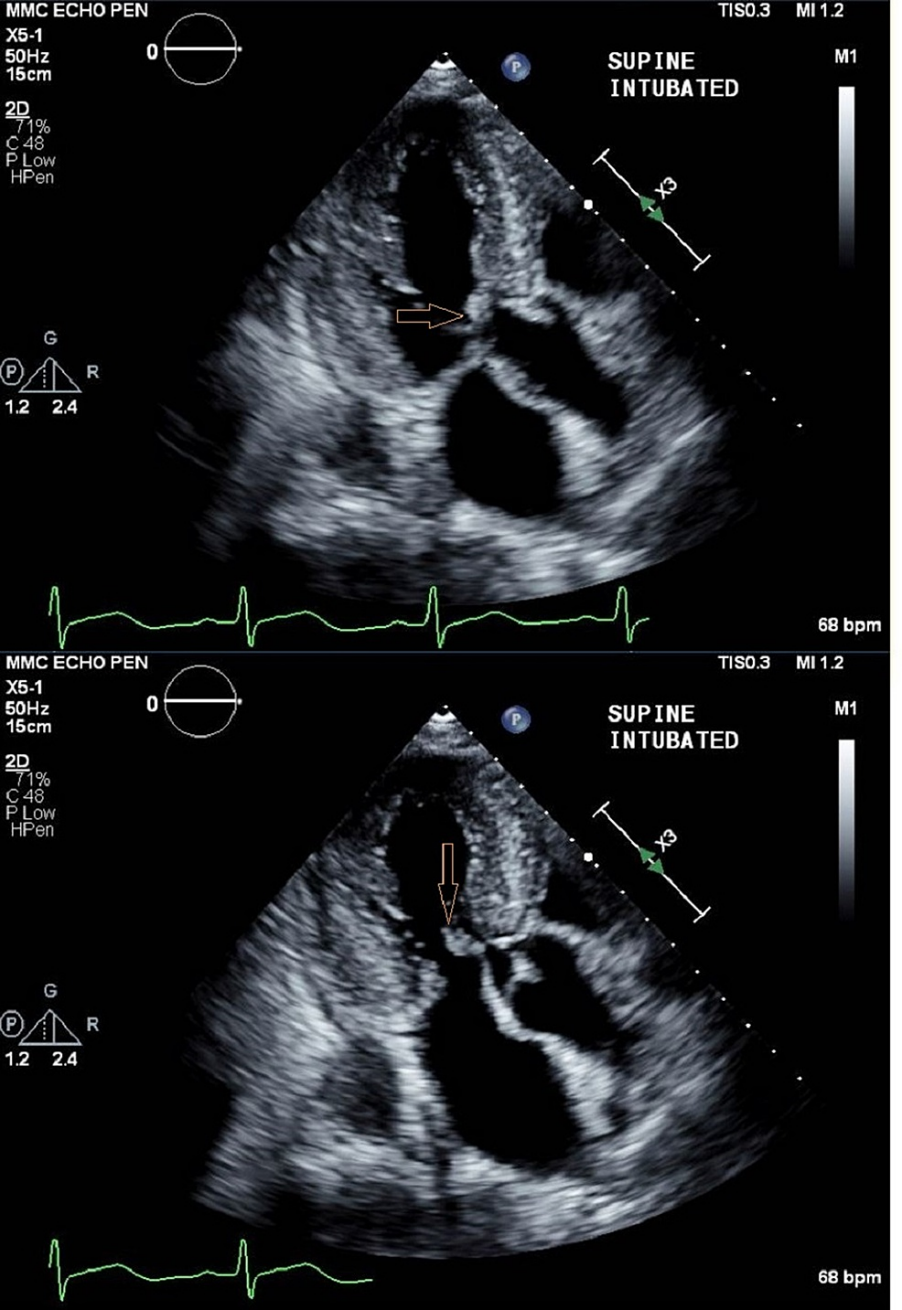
Transthoracic Echocardiogram Transthoracic echocardiogram obtained postoperatively to highlight the extent of left ventricular outflow tract obstruction. In the bottom image, the anterior mitral valve leaflet is highlighted during diastole. In the top image, the anterior mitral valve leaflet is displaced into the left ventricular outflow tract, resulting in restriction of forward blood flow.
